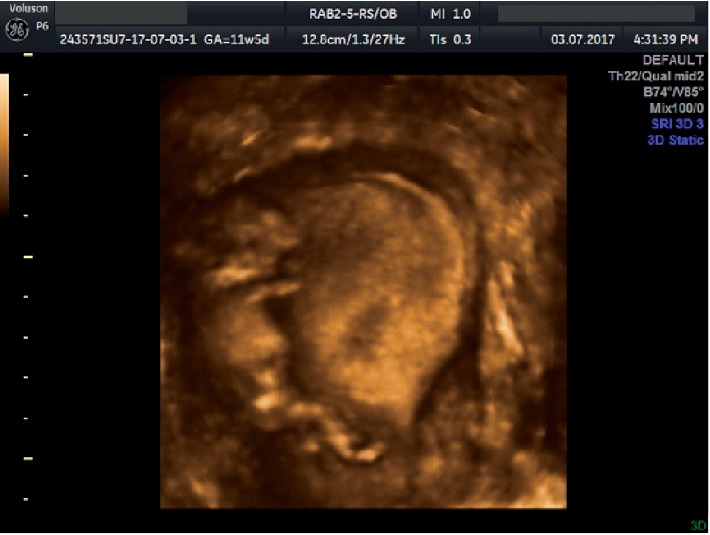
First trimester ultrasound: the concurrent presence of fetus and fetal bone.
